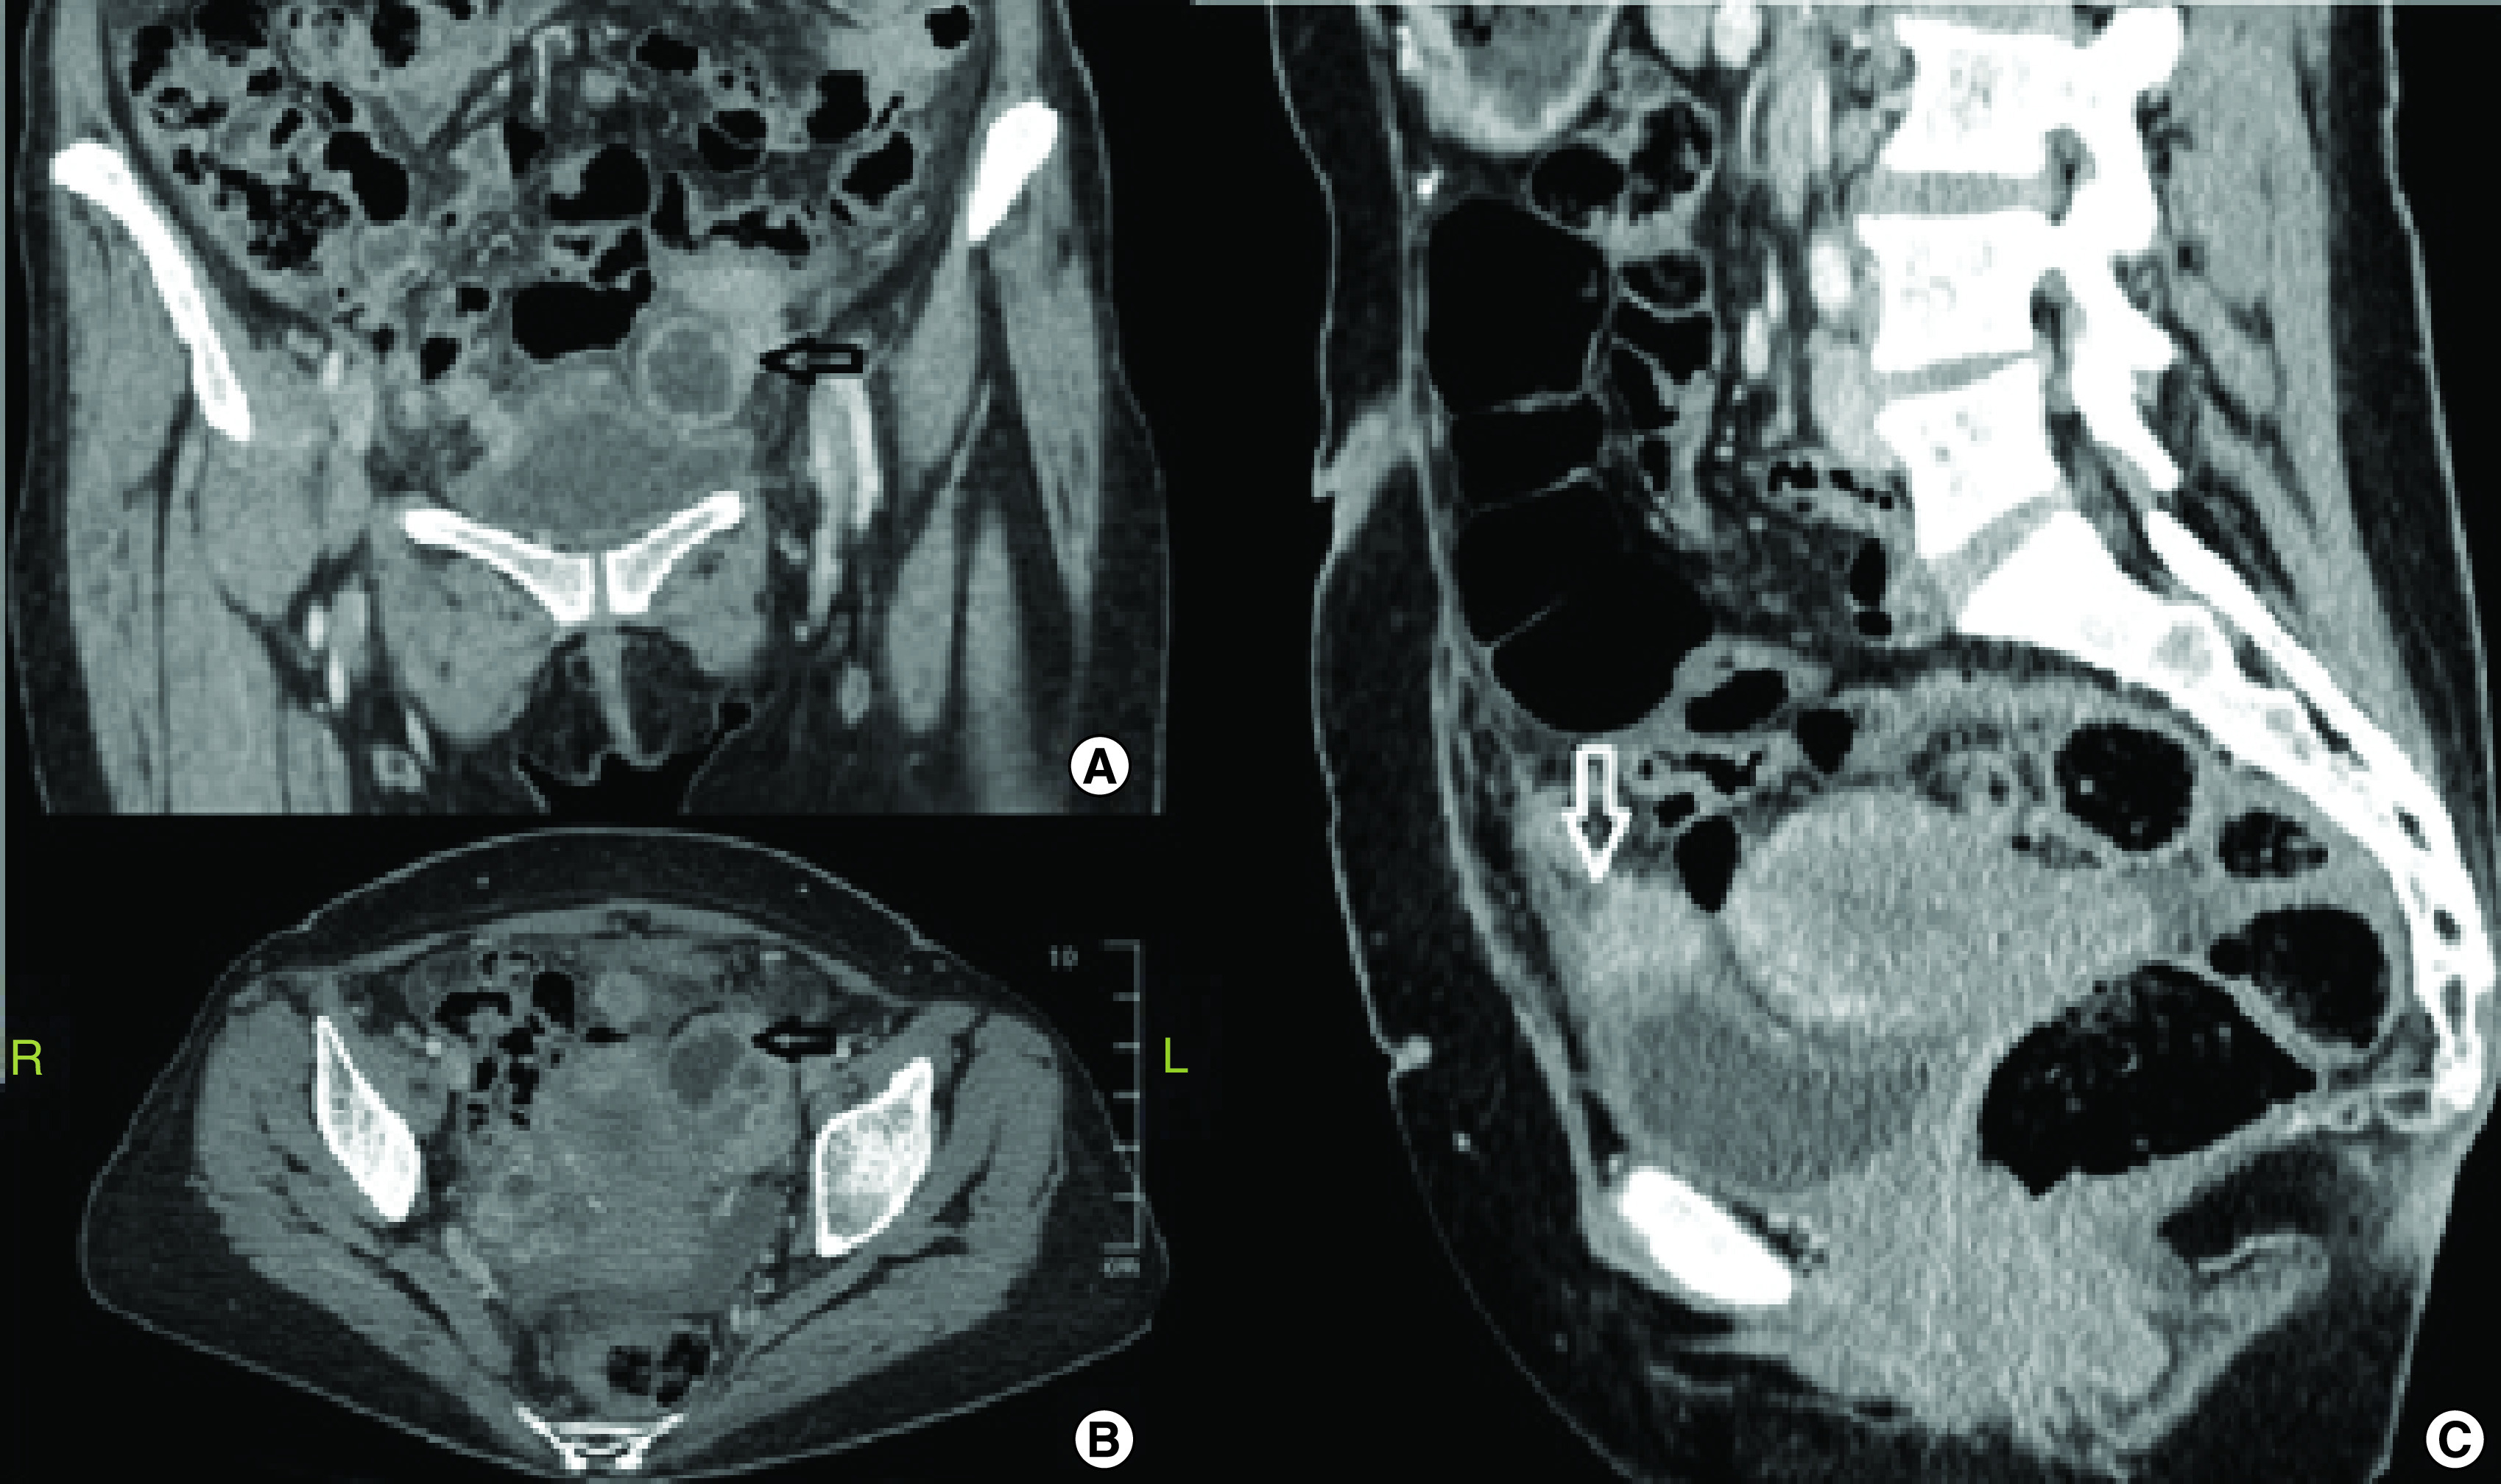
Pelvic computed tomography scan images during first emergency admission. (A & B) Contrast enhanced computed tomography showing the conglomerated mass around the left adnexa filling the pelvis on coronal and axial views. (C) Enhanced 2 cm implant lying on the bladder dome and infiltrating its wall with a bulging effect.
